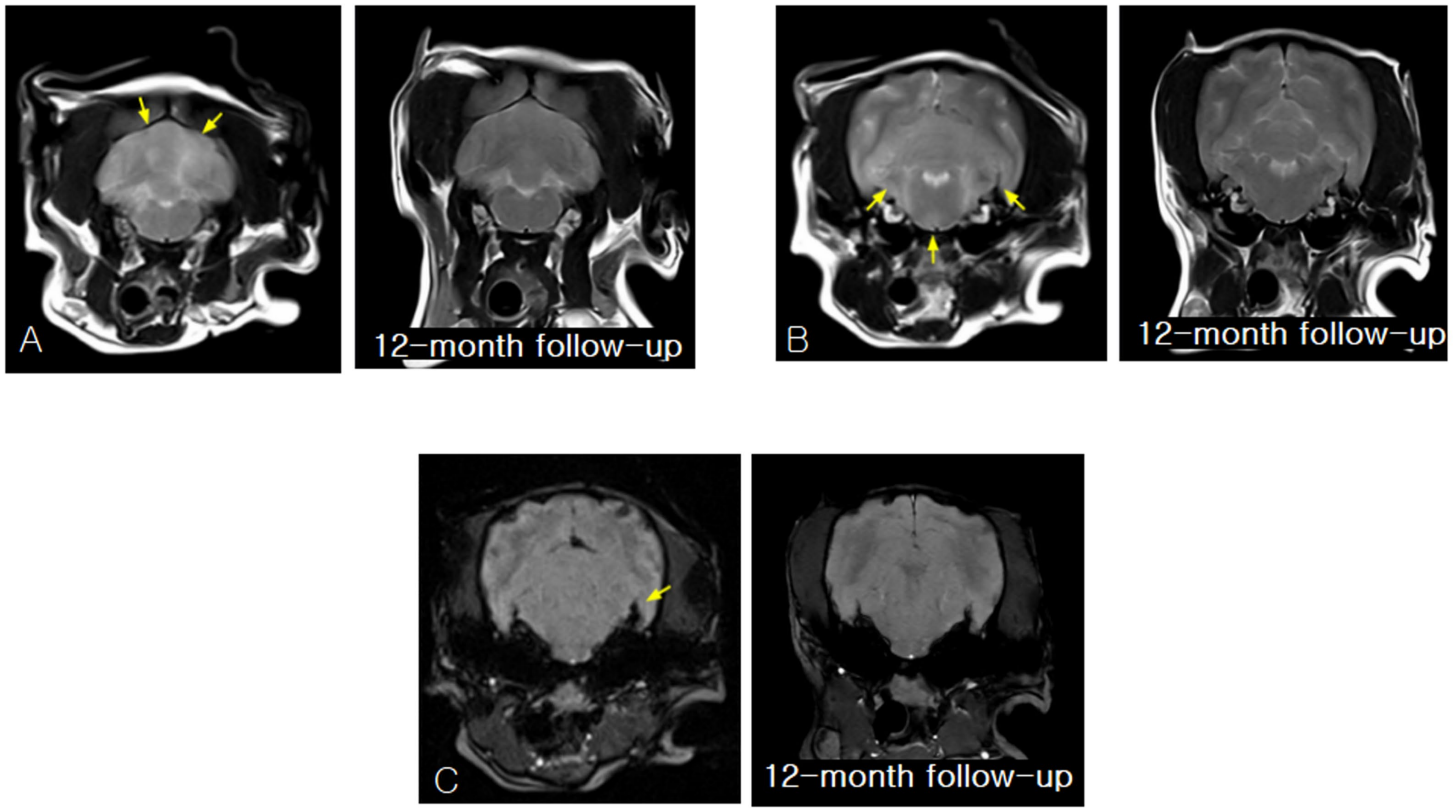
Initial and 12-month follow-up magnetic resonance imaging (MRI) findings obtained after initiation of steroid-free immunosuppressive therapy, demonstrating resolution of all previously identified inflammatory lesions. (A) Baseline transverse T2-weighted image showing bilateral, poorly marginated hyperintensity within the cerebellar hemispheres, consistent with diffuse intra-parenchymal inflammatory change. At the 12-month follow-up, a transverse T2-weighted image obtained at the same anatomical level demonstrates normalization of cerebellar parenchymal signal intensity, with resolution of the previously observed T2 hyperintensities and restoration of normal gray–white matter distinction. (B) Baseline transverse T2-weighted image demonstrating ill-defined, bilateral hyperintense lesions involving the pons and medulla, consistent with non-mass-like inflammatory infiltration of the caudal brainstem. At the 12-month follow-up, a transverse T2-weighted image obtained at the same anatomical level shows resolution of the previously identified bilateral T2 hyperintense lesions, with restoration of a uniform and symmetrical brainstem parenchymal signal and no evidence of residual inflammation. (C) Baseline susceptibility-weighted imaging (SWI) revealing an asymmetric hypointense focus within the left pontine region, suggestive of localized susceptibility changes compatible with active inflammatory disease. At the 12-month follow-up, SWI obtained at the same level demonstrates disappearance of the previously observed hypointense focus, with no residual susceptibility artifact, indicating resolution of the inflammatory-associated signal change.
